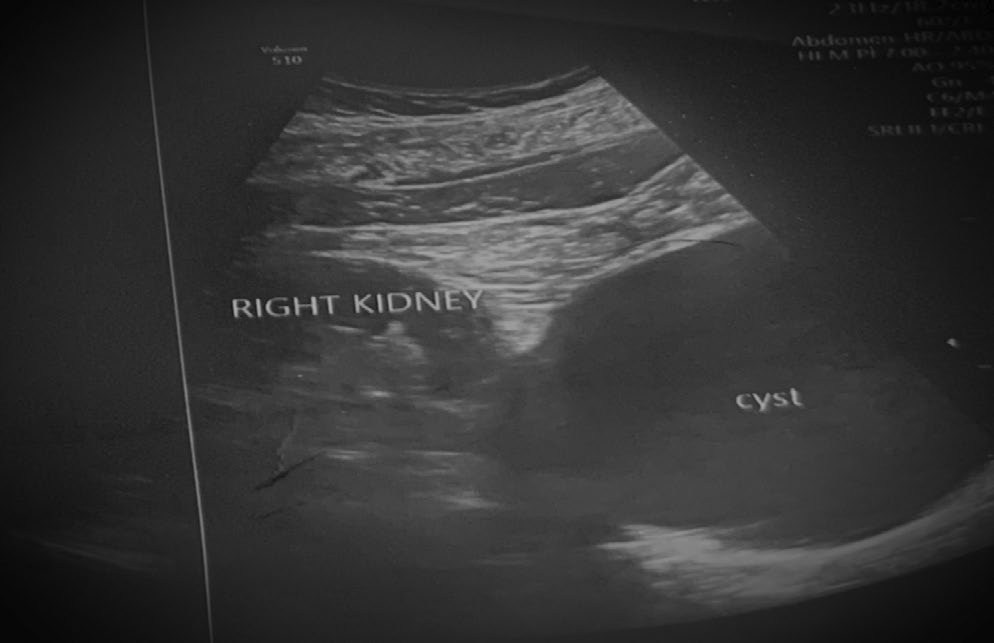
Ultrasound of abdomen showing cyst on lower pole of right kidney.
